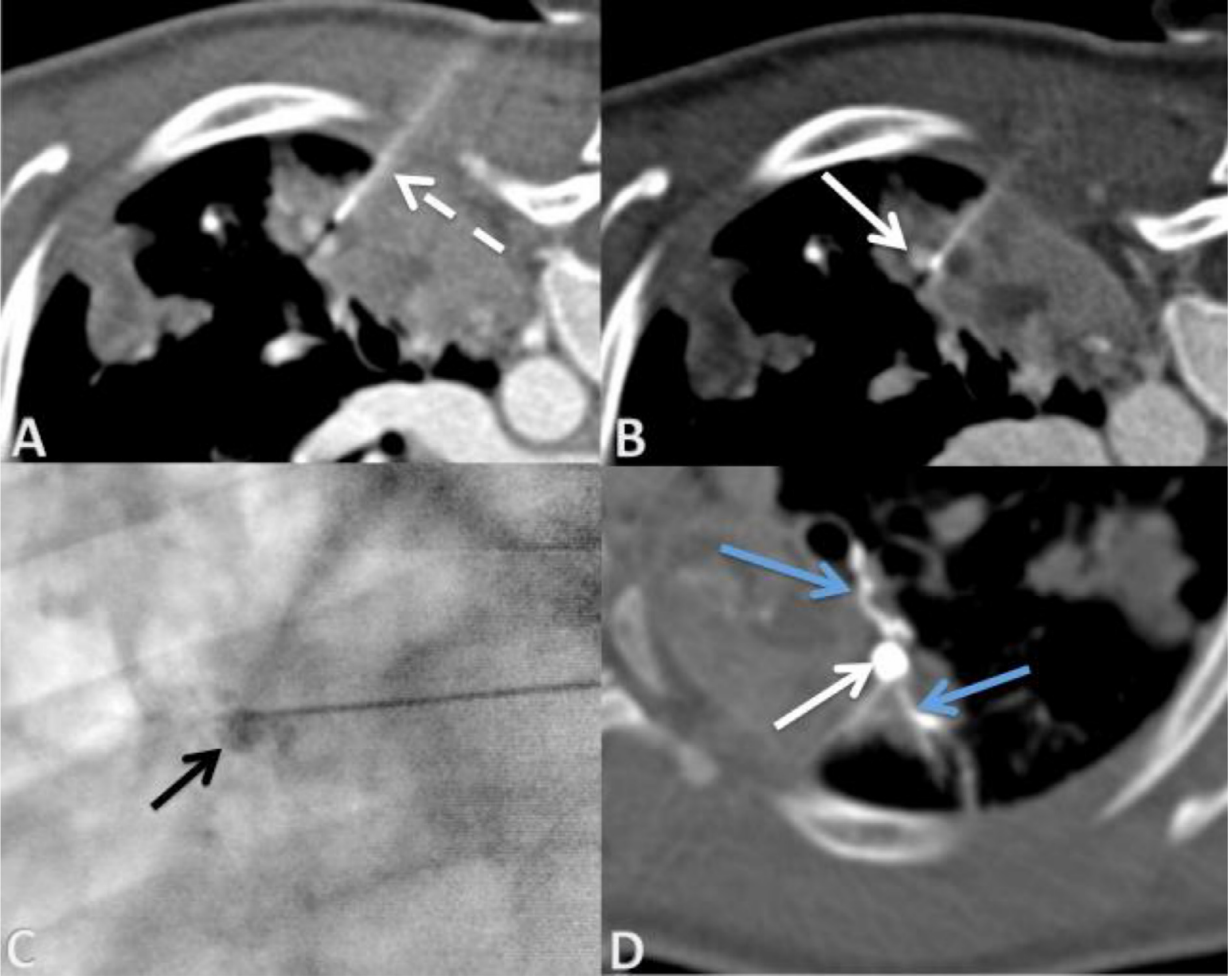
(A) After a CT scan angiogram, in procubitus, a 22G needle is inserted into the parenchyma, perpendicularly to the pleural surface, through the parenchymal condensation, in order to reduce the risk of pneumothorax (dotted arrow). (B) The per-procedure CT scan shows the correct positioning of the needle within the PAP (white arrow). (C) Scopic examination shows complete filling of the PAP (black arrow) after injection of a 0.5 cc mixture of Glubran2/Lipiodol 1/1. (D) The final CT scan demonstrates complete filling of the pseudoaneurysm (white arrow) and the artery feeding it (blue arrows). (Coloured version of the figure is available online).
